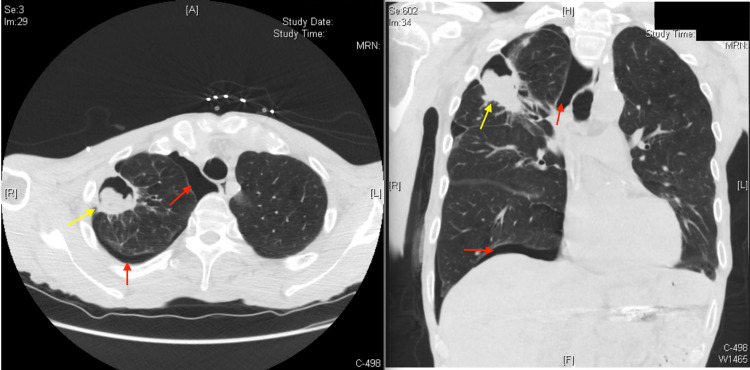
CT chest with IV contrast (axial reformatted: left image) (coronal reformatted: right image) demonstrating right-sided pneumothorax (red arrows) and right-sided apical cavitary lesion (yellow arrow). CT: computed tomography; IV: intravenous
